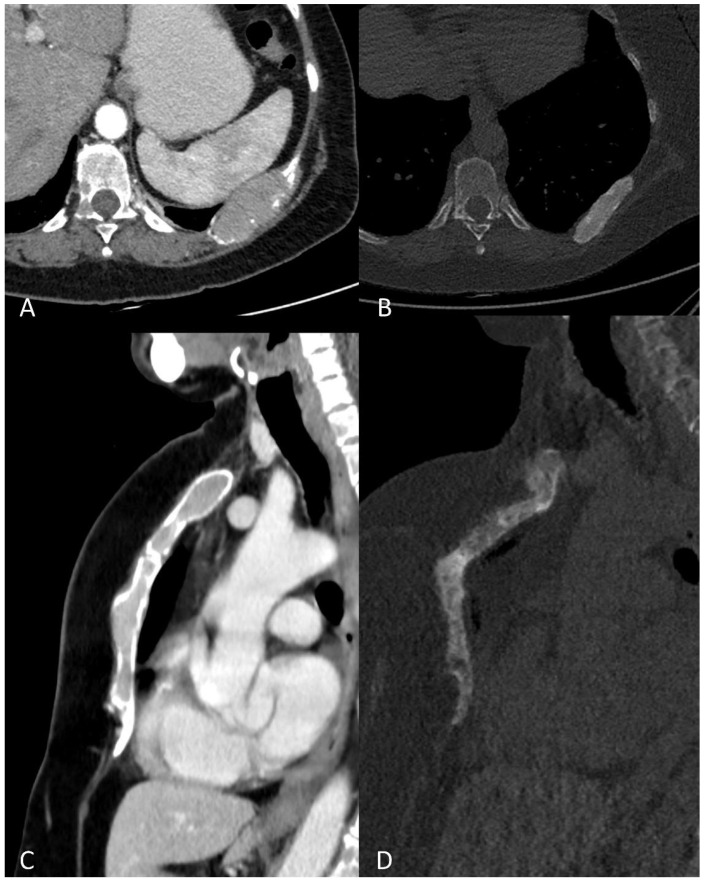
Multiplanar CT images showing (A) an osteolytic lesion of a rib, with (B) development of sclerosis and size-reduction after treatment. (C) Diffuse hyperdense myeloma deposits in the medullary cavities of the sternum with (D) development of sclerosis and size-reduction after treatment.
